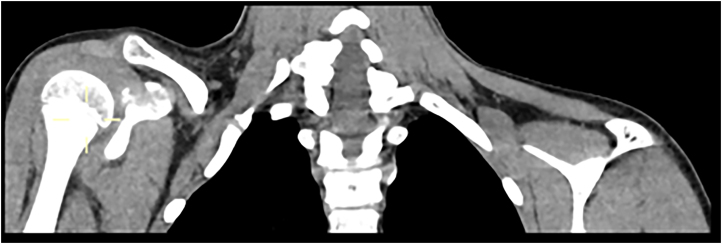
Coronal computed tomography view of the clavicle to show the extent of compression on surrounding structures
